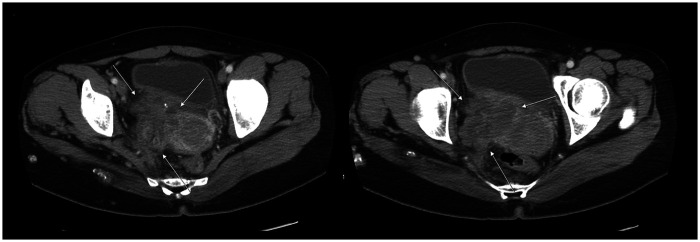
Pre-treatment contrast-enhanced abdominopelvic CT (APCT) images. Pre-treatment APCT demonstrated a 7.5 cm vascular lesion in the right pelvic cavity abutting the right side of the vagina, uterus, adnexa, bladder, and pelvic sidewall and encasing the right internal iliac chain. The lesion was associated with mild right hydronephrosis, likely due to a distal ureteral obstruction. In addition, multifocal enhancing nodular lesions were observed on the right buttocks and thigh.
